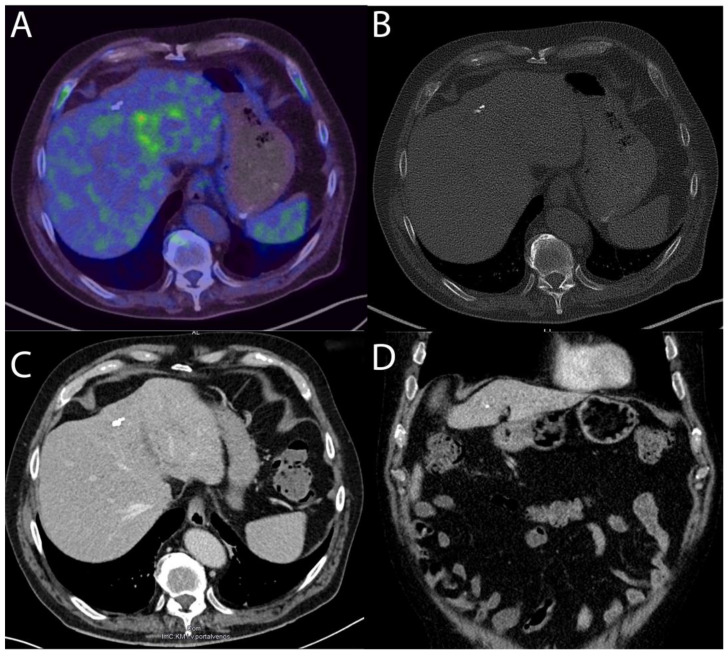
Follow-up imaging for staging. (A): PET-CT with delayed acquisition after 3 h showing a polyform ^18^F-FDG enhancement in projection onto the irregular hypodense lesions with an SUV of max. 5.45 (captured with Siemens Biograph 40/64 True Point, Siemens, Munich, Germany). (B): Native CT performed as part of the FDG-PET-CT showing slight micro and macro calcifications. (C,D): Contrast-enhanced CT using Accupaque, captured in axial and frontal planes with a Siemens SOMATOM Definition Flash, demonstrating persistent calcifications and regression of the focal hypodense lesion.
